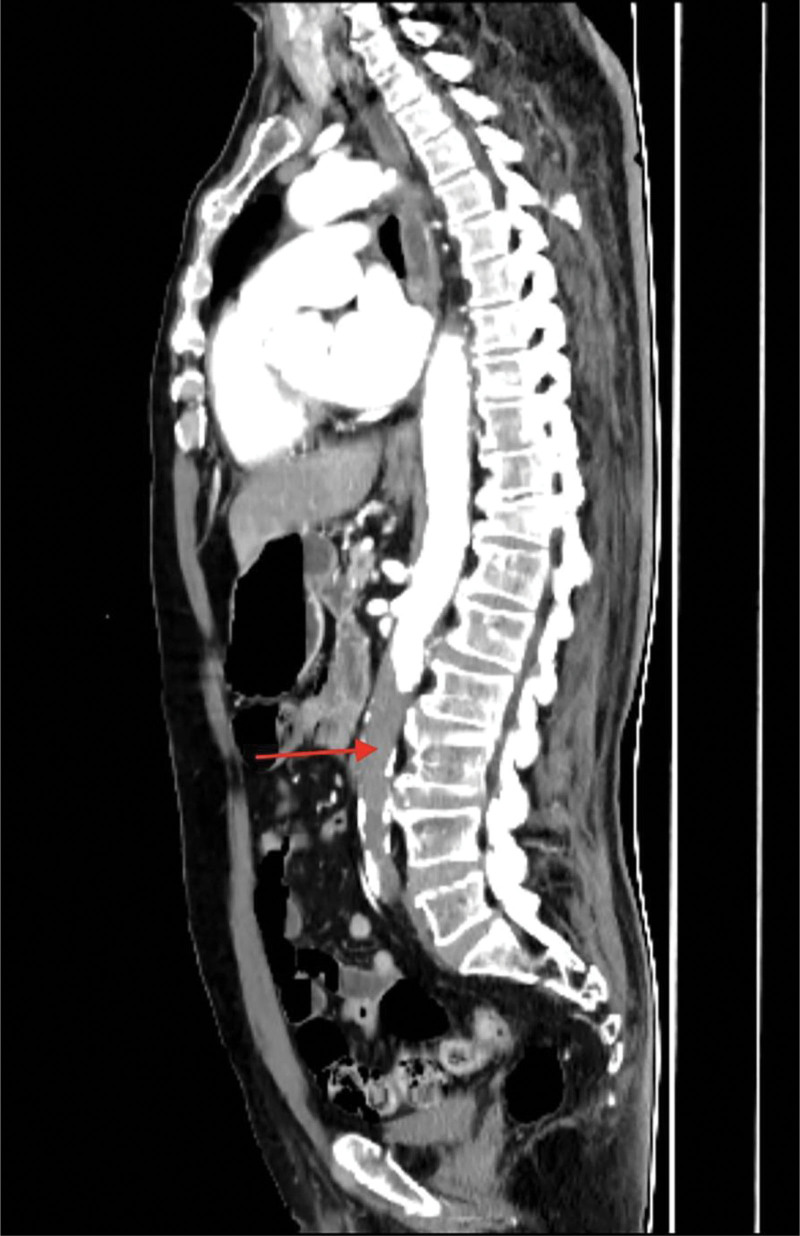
Computed tomography angiography of the chest and abdomen (sagittal view) demonstrates occlusion of the descending aorta.
